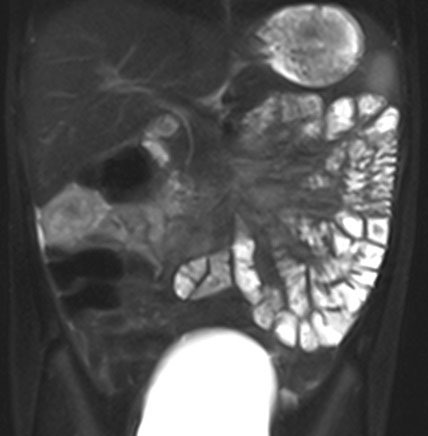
Figure 2: The fat suppressed T2W MRI sequence confirms the presence of fat within the lesion as shown by the arrow.
